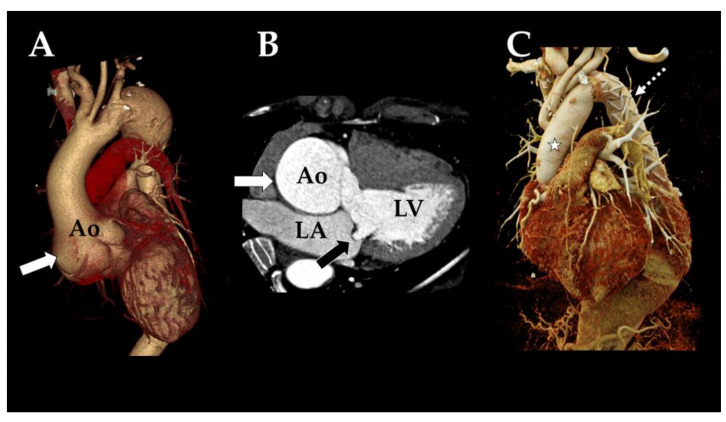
Thoracic aorta CTA findings in Marfan syndrome patients: The 3D volume rendering technique image (A) and three-chamber view image (B) showed dilated aortic root in tulip bulb configuration and annuloaortic ectasia (white arrow). Mitral valve prolapse is demonstrated (B: black arrow). The post-operative CTA image demonstrated the normal size of the vascular graft at ascending thoracic aorta (C: star) and evidence of thoracic endovascular aortic repair (C: dashed arrow). (Ao; aorta, LA; left atrium, LV; left ventricle).
